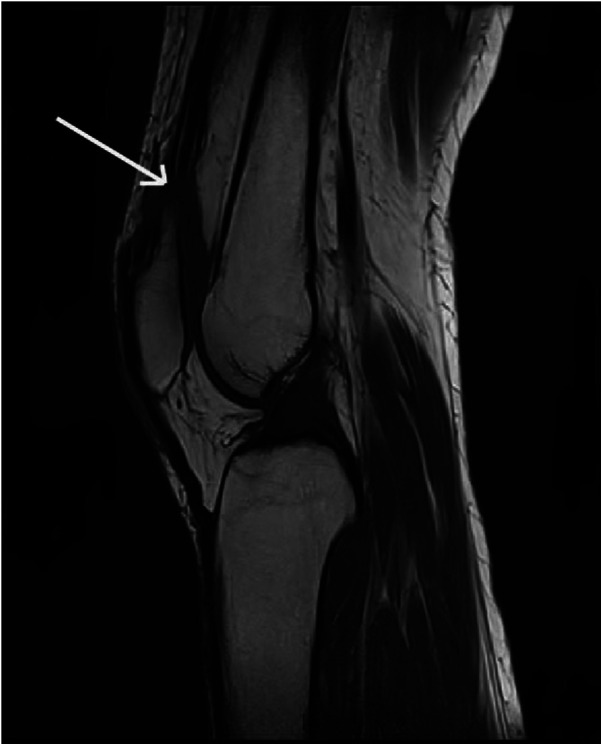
Preoperative MRI of the knee showed rupture of the quadriceps femoris tendon.
